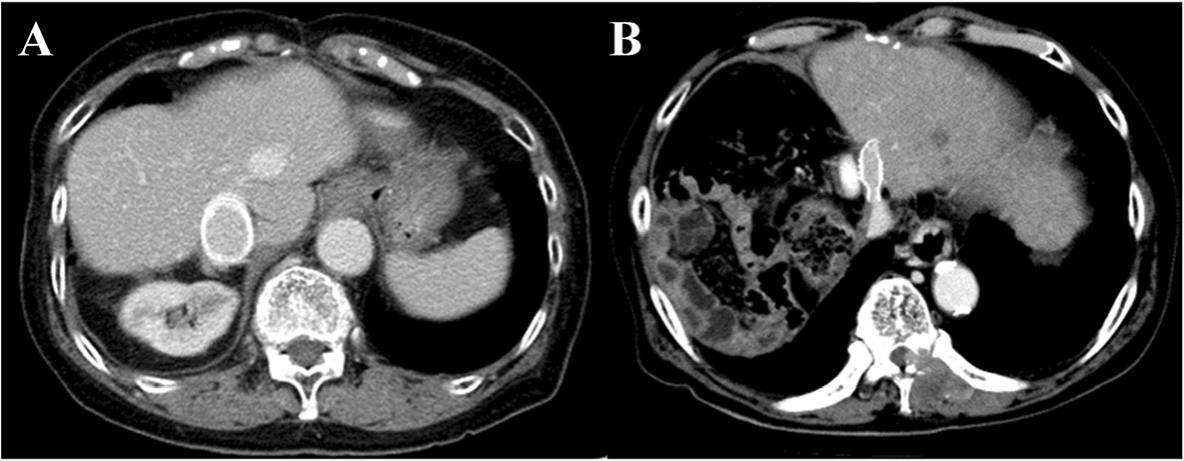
Postoperative enhanced computed tomography. (A) The patency of an inferior vena cava graft has been kept for nine years after the operation (patient 3). (B) The patency of a hepatic vein graft has been kept for one year after the operation (patient 4).
